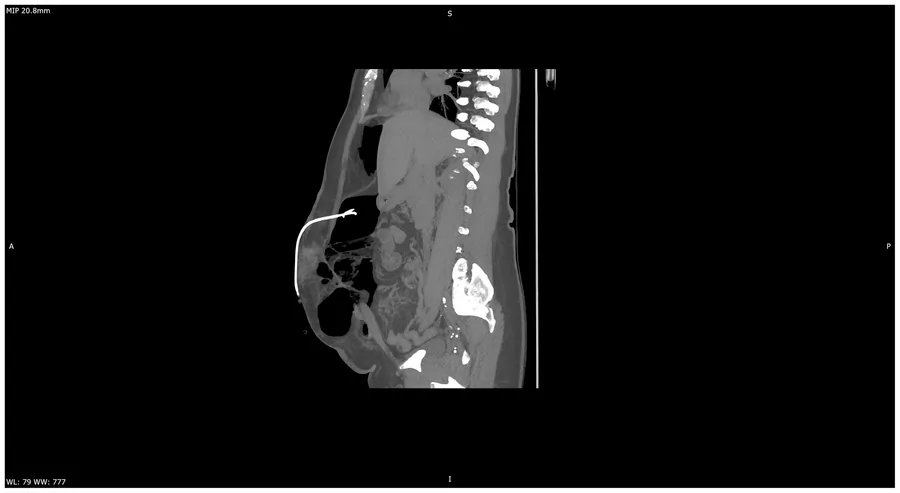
CT-guided progressive preoperative pneumoperitoneum (PPP). Sagittal CT image demonstrating the intraperitoneal catheter following successful placement and expansion of the abdominal cavity with ambient air during the PPP protocol.
